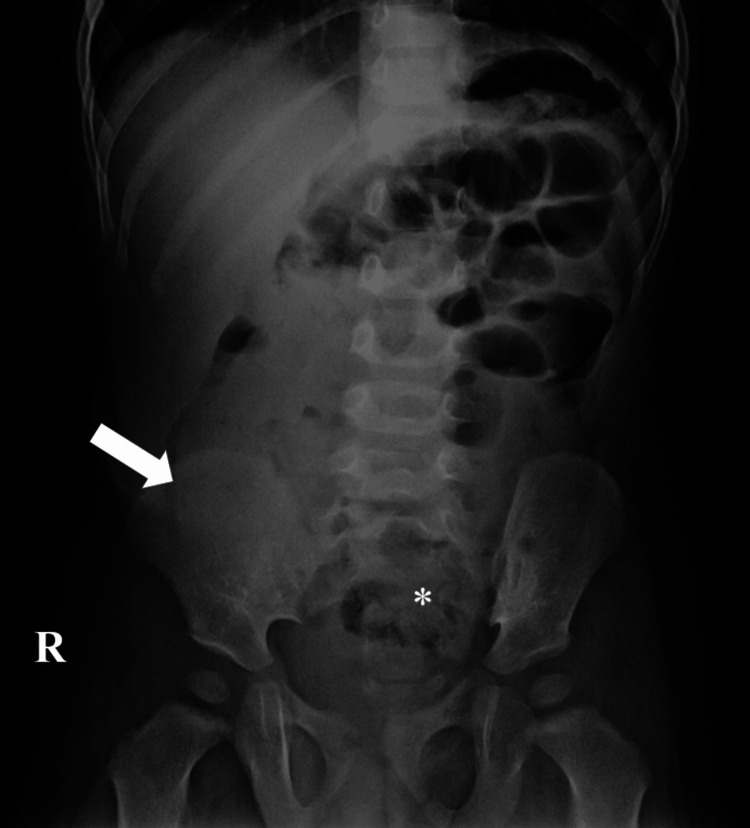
Plain X-ray of the abdomen revealing soft-tissue mass in the RUQ and RLQ Abdominal radiography demonstrates increased radiopacity, especially in the RLQ (arrow), extending toward the hepatic flexure and proximal transverse colon. Distal air in the abdomen is detected (asterisk) R: right; RLQ: right lower quadrant; RUQ: right upper quadrant
